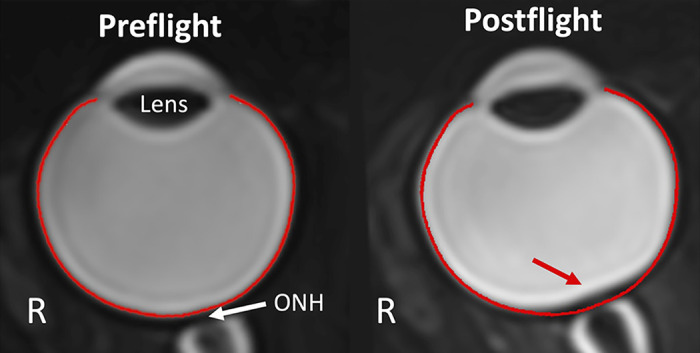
Posterior globe flattening near the optic nerve head (ONH) has been identified subjectively and objectively after long-duration spaceflight and as a major symptom of spaceflight associated neuro-ocular syndrome (SANS). A contour (red) is overlayed on a preflight and postflight T2-weighted MRI to show globe flattening that occurred after long-duration spaceflight that corresponds to a volume displacement of 39.2 mm^3^. This image was obtained as a part of the NASA Ocular Health Study (7).
